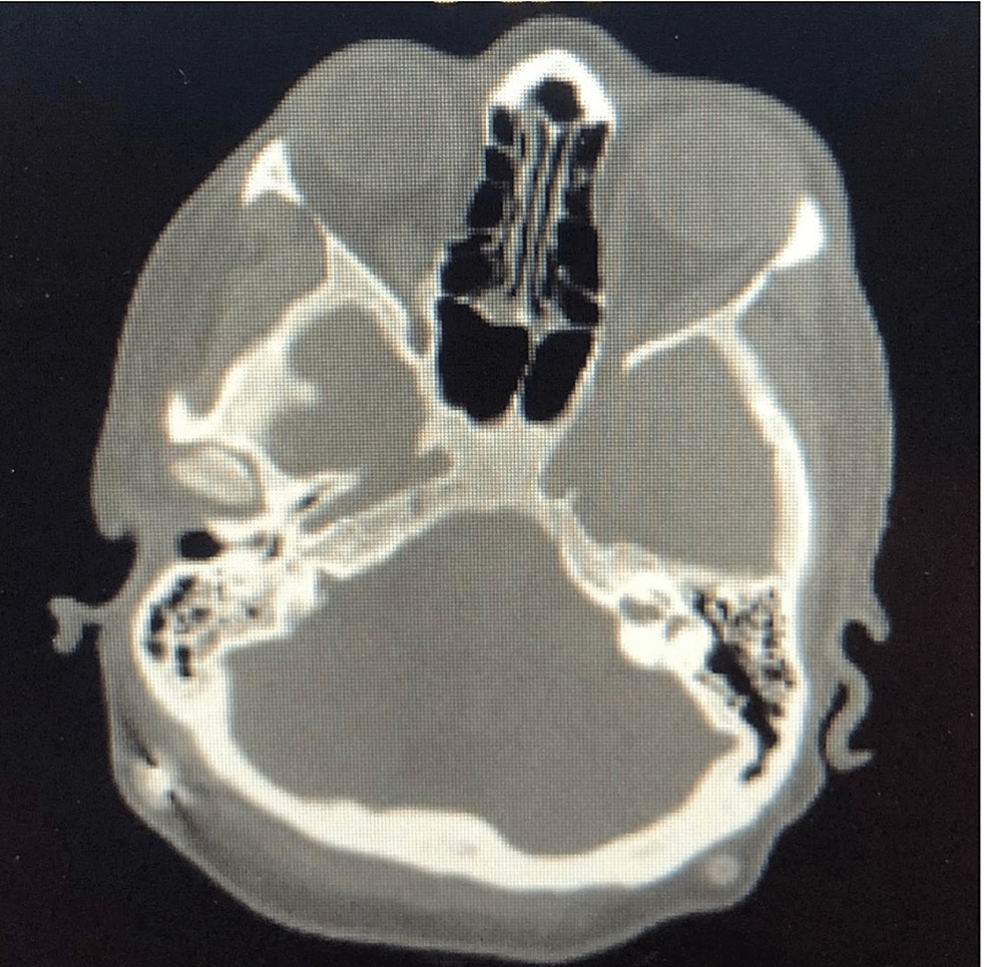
CT of the head confirming chronic subdural hematomas with no acute process A head CT was repeated on 04/06/2020, 04/14/2020, and 05/11/2020 with no reported change in the impression. The above image is from 04/06/2020. Impression: No acute intracranial pathology, no abnormal enhancement, stable bilateral ventriculostomy catheters as described, small chronic bilateral subdural hematomas measuring up to 0.4 cm in depth CT: computed tomography
